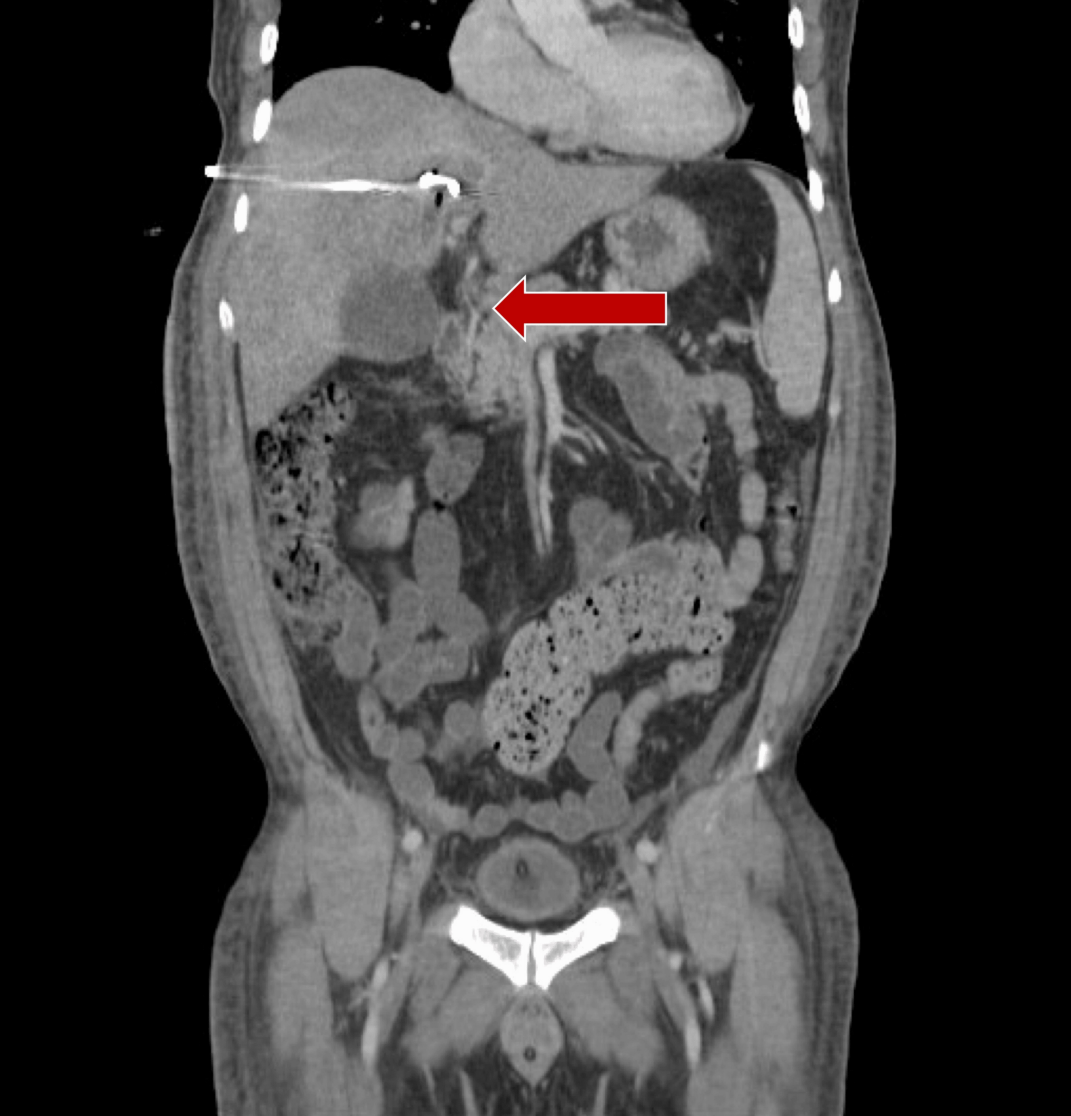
Control CT scan after the second percutaneous drainage Reduction of the liver mass to 31 × 29 × 37 mm, located in segments IVa, IVb, VI and VII, and with drainage in correct position.
